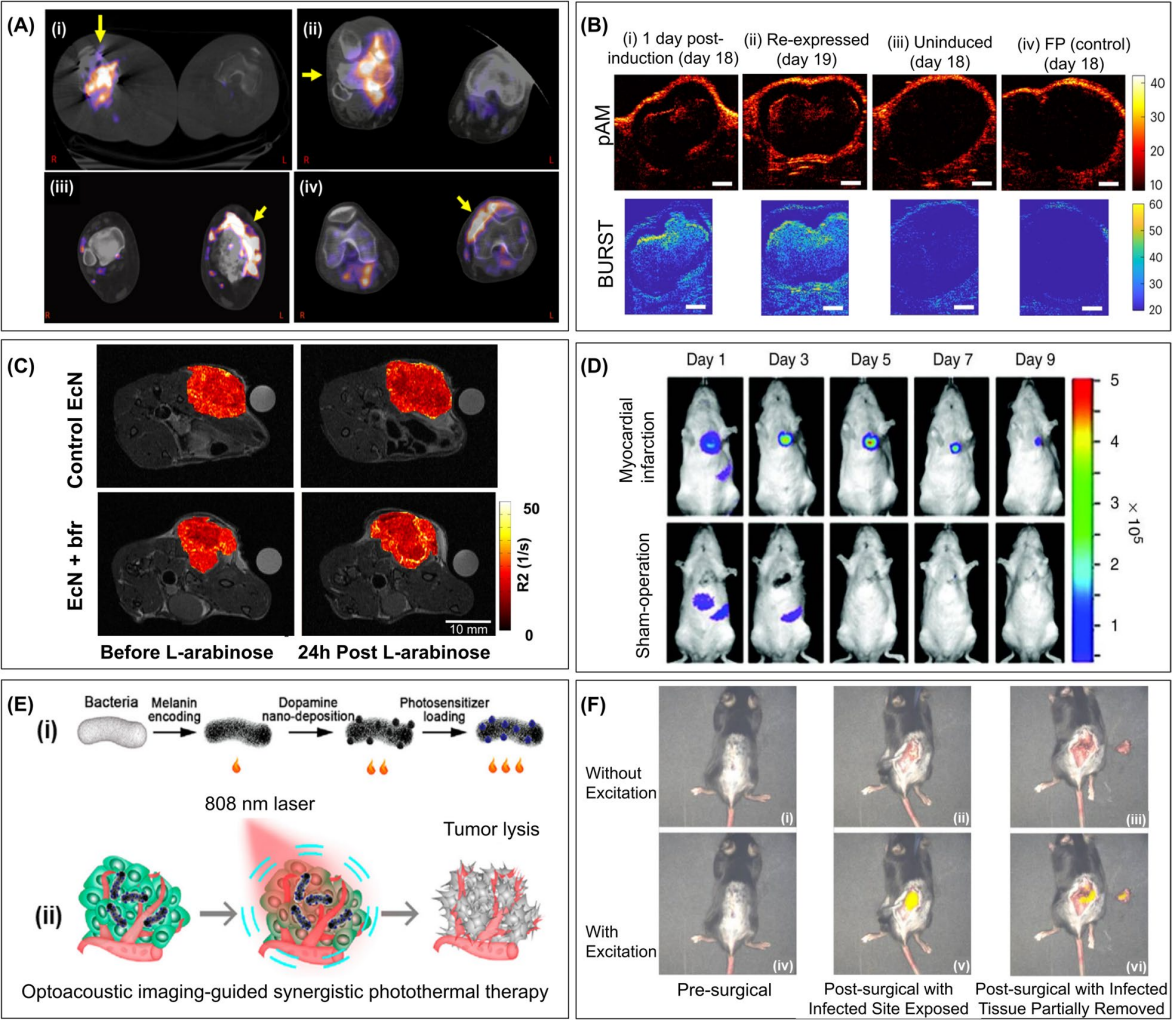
Bacteria as imaging vectors. A Super-imposed PET and CT images of established infection 2 h post [^124^I]FIAU radiotracer administration in human patients. (i) methicillin-resistant *Staphylococcus aureus* (MRSA)-caused septic arthritis (right knee with a prosthetic joint), (ii) MRSA-induced septic arthritis (right knee with history of chronic MRSA osteomyelitis), (iii) cellulitis (left lower extremity with history of osteomyelitis in the left distal tibia), (iv) necrotizing MRSA osteomyelitis of the proximal tibia (left knee). Reprinted with permission from [130]. B In situ bARG_Ser_ expression by *E. coli* Nissle 1917 (EcN) for ultrasound imaging of tumor colonization. Representative parabolic ultrasound (pAM) and Burst Ultrasound Reconstructed with Signal Templates (BURST) images of tumors colonized by bARG_Ser_-expressing EcN (i) at 1 day after induction with intraperitoneally injected L-arabinose (on day 18 post subcutaneous injection of tumor cells and day 4 post tail vein injection of EcN bacteria), (ii) at 1 day after the collapse of gas vesicles and re-injection of L-arabinose for re-induction (day 19), (iii) uninduced on day 18, (iv) pAM and BURST images of tumors colonized by fluorescence protein-expressing (FP) EcN after L-arabinose induction. Reprinted with permission from [135]. C
